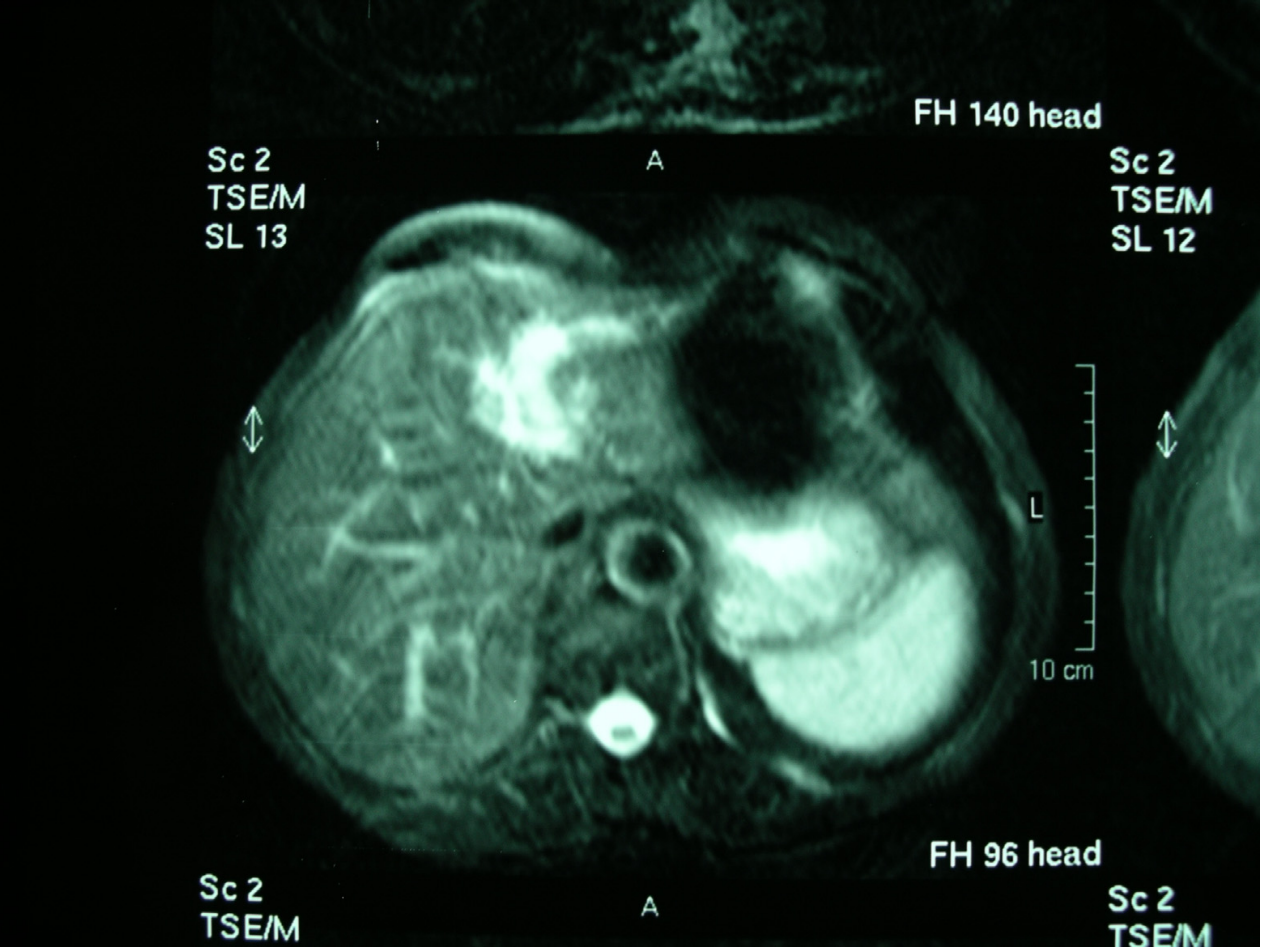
Abdominal magnetic resonance imaging demonstrating the dilated left hepatic duct, without the presence of any space-occupying lesion.
